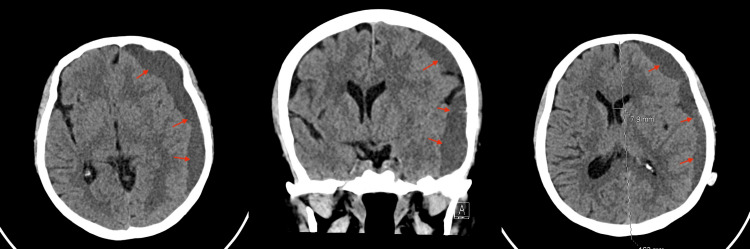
A total of 18-day brain CT post-MMA embolization. There is an interval increase in the size of the left cerebral convexity subdural hematoma. The maximum thickness measures approximately 2 cm, causing a mass effect, more prominent on the left temporal lobes. There is also an interval worsening of the midline shift to the right side, now measuring approximately 8 mm. MMA: Middle meningeal artery.
